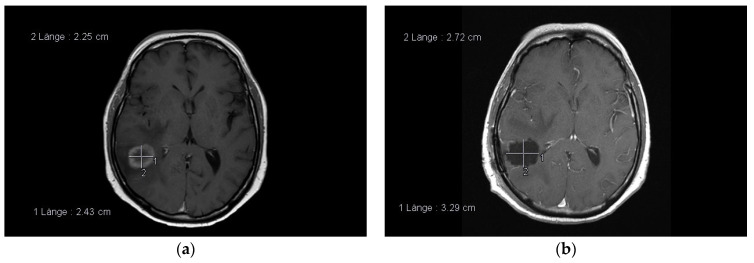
(a,b): Examples of circumferential stripping. Adenocarcinoma metastasis in the right temporal lobe, note the resection cavity diameter exceeds the diameter of the contrast-enhanced lesion (pre- and postoperative. MRI, T1, contrast-enhanced).
